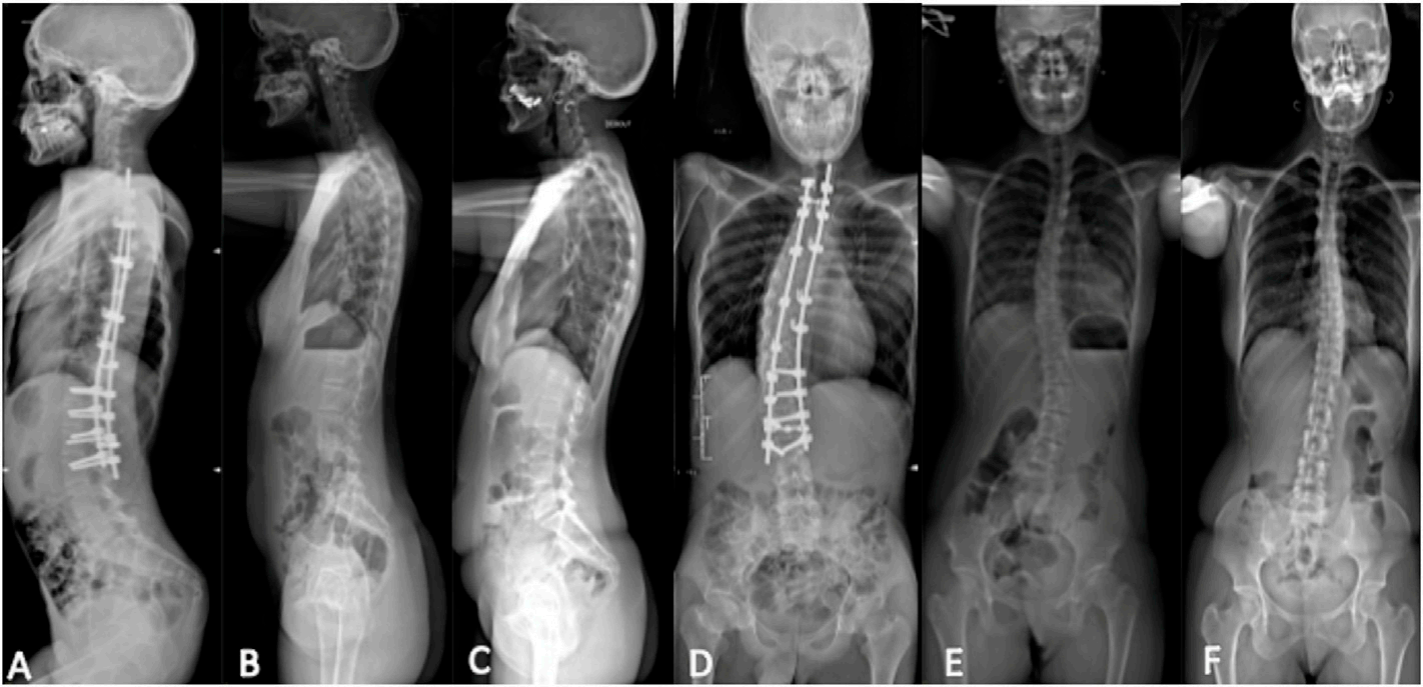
Spinal X-ray using the EOS technique. Lateral view: (A) index case, (B) sister, and (C) mother; posteroanterior view: (D) index case, (E) sister, and (F) mother. Thoracolumbar scoliosis with right convexity was observed from the atlanto-axial junction to L3 in the index case. X-ray showed thoracolumbar scoliosis with left convexity in the mother and sister.
